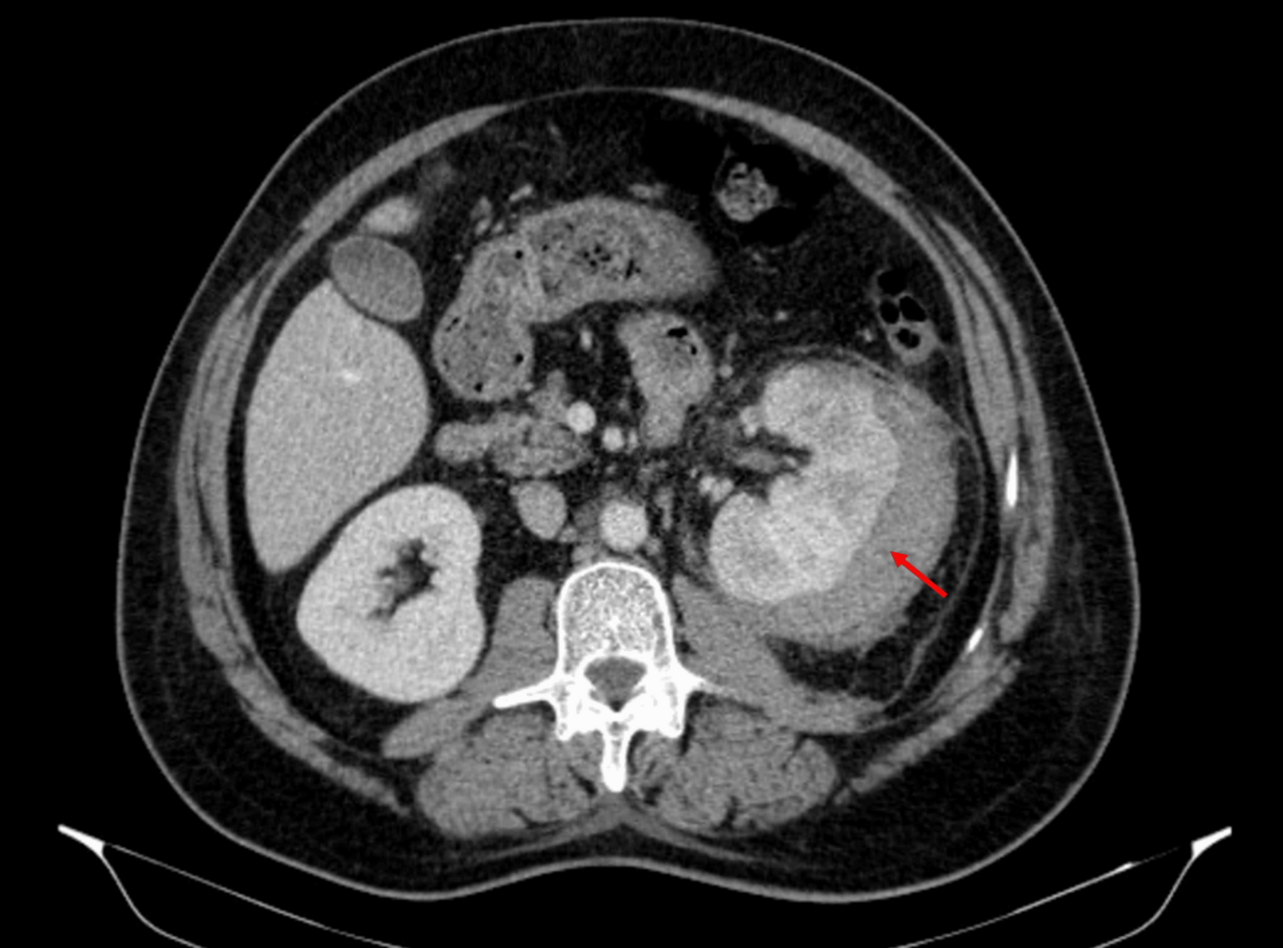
Contrast-enhanced abdominal CT scan shows a left external subcapsular collection, consistent with a subcapsular hematoma (red arrow). No abnormal vascular malformations or tumors were found.
